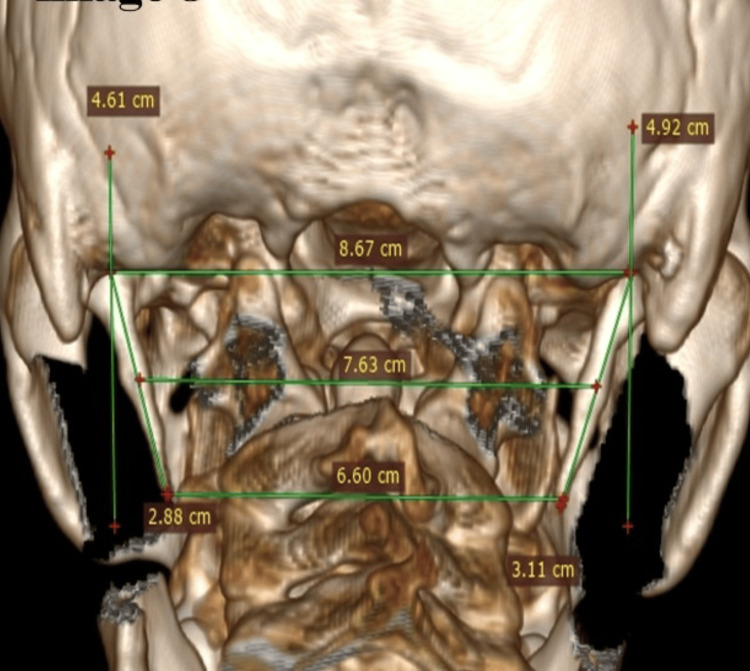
Unilaterally (>3 cm) elongated styloid process on posterior view of 3D CT. 3D CT, three-dimensional computed tomography.
